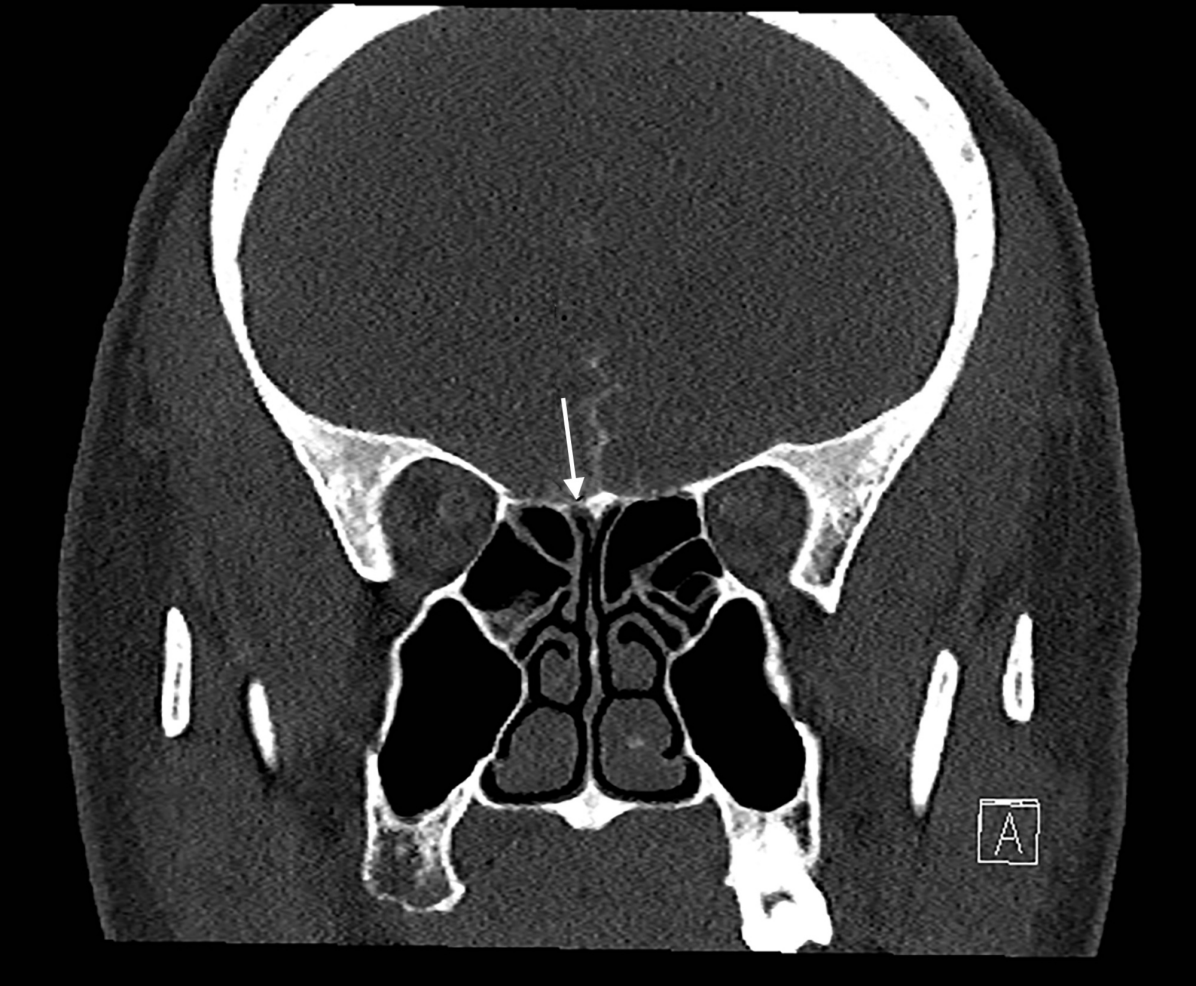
CT of the cribriform plate, coronal view Computed tomography (CT) reveals a severe thinning of the cribriform plate and fovea ethmoidalis bilaterally, the right worse than the left with multiple suspected small focal regions of cortical discontinuity along the right cribriform plate and fovea ethmoidalis.
